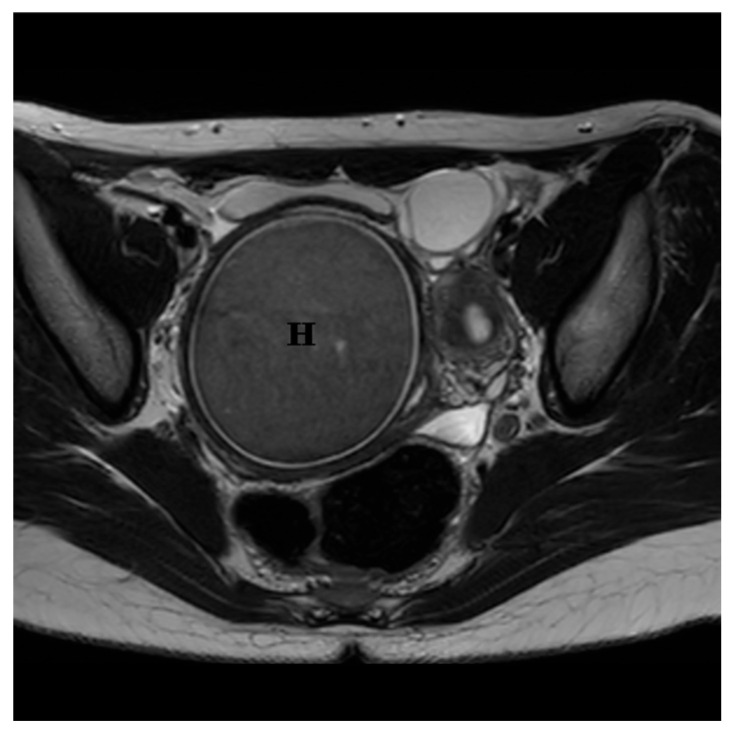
Axial T2-weighted MRI, obtained prior to hematocolpos incision, demonstrated a well-circumscribed, homogeneously hypointense collection within the right hemivagina, consistent with hematocolpos (H). The image also demonstrated an associated Müllerian duct anomaly classified as U3C2V2 (uterus didelphys with duplicated cervices and vaginas). Both ovaries were visualized and appeared unremarkable, without focal lesions. No lymphadenopathy or increased free pelvic fluid was observed.
